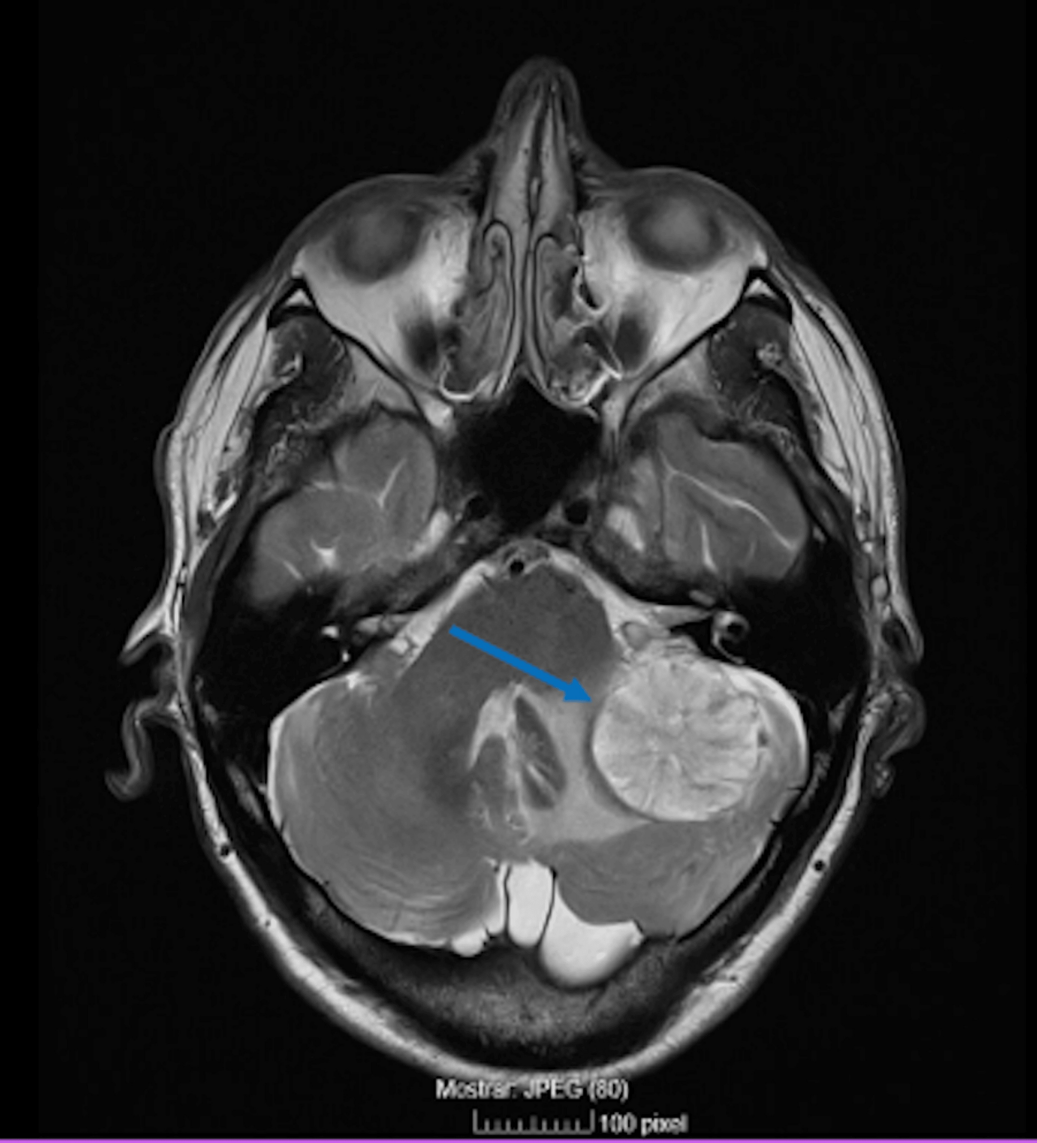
Brain MRI showing an intra-axial expansive lesion in the left posterior fossa measuring 37 × 37 × 35 mm
